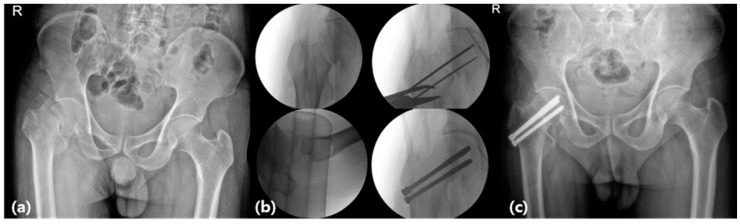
Operation for a femoral neck fracture. (a) Preoperative X-ray (anteroposterior view of both hips), (b) intraoperative fluoroscopy, and (c) closed reduction and internal fixation (cannulated screw). Abbreviation: R, right side
